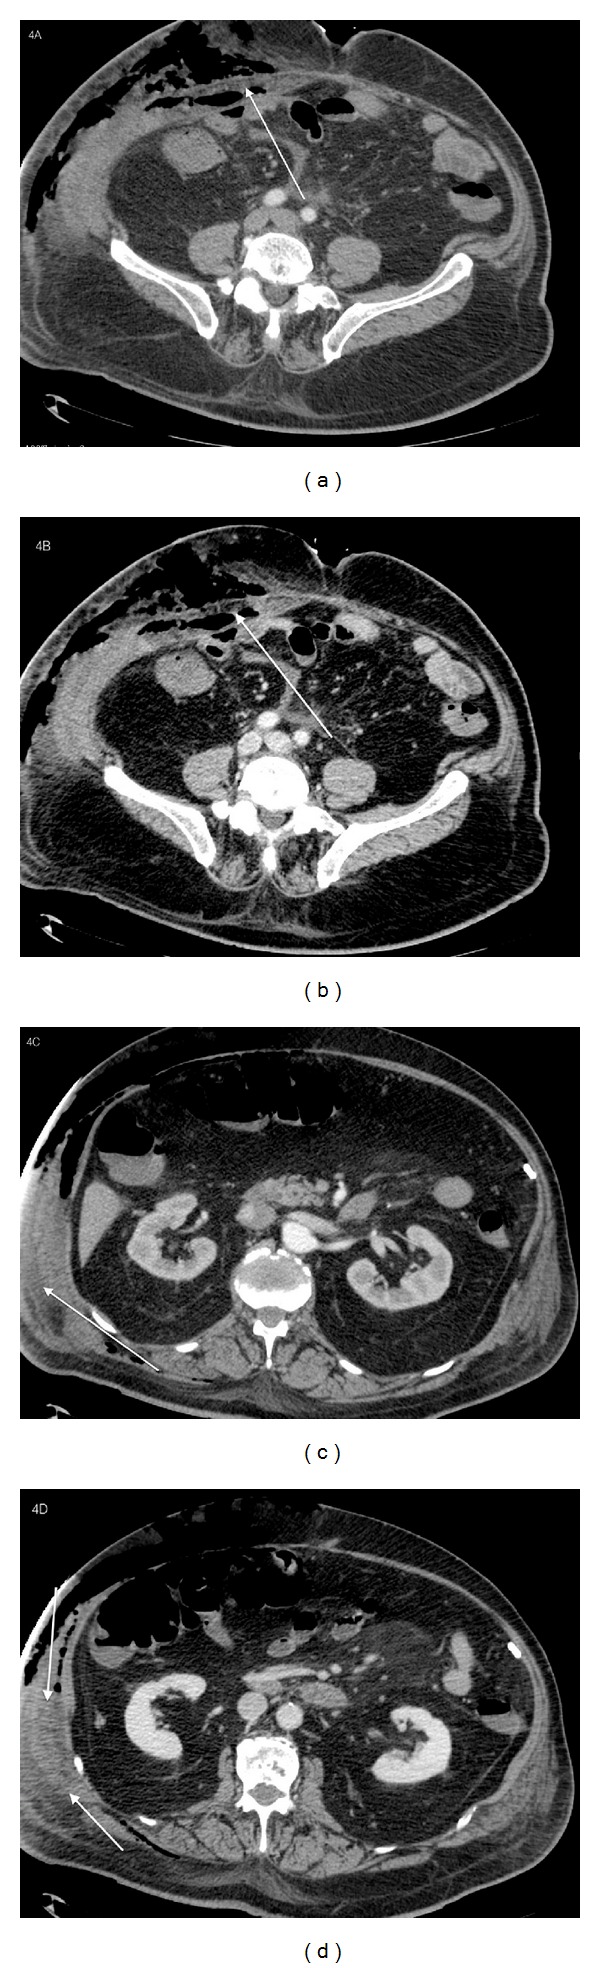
78-year old male with diagnosis of postsurgical necrotizing fasciitis. Postcontrast arterial and early venous phase, axial CT. After the administration of the contrast medium the fascia appeared as follows: not enhanced in the arterial phase (a) and weakly enhanced in the early venous phase (c), and areas of low attenuation ((c) and (d)) within the muscles and among the fat tissues structures of the right flank were observed. (a) Axial arterial phase, the fascia appeared not enhanced. (b) Axial early venous phase, the fascia appeared weakly enhanced. (c) Axial arterial phase shows the presence of areas of low attenuation among the muscles of the right flank. (d) Axial early venous phase shows the presence of areas of low attenuation among the muscles of the right flank.
